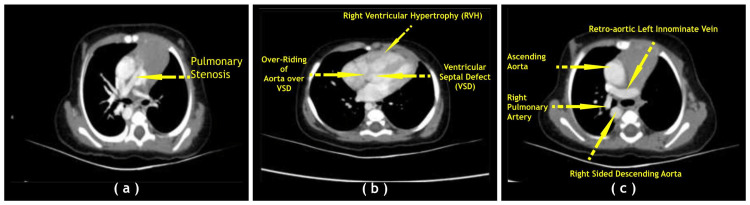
(a) CT axial image showing pulmonary stenosis and hypoplastic pulmonary arteries in a 6-month-old baby, who underwent primary Brock’s operation; (b) Axial image showing ventricular septal defect with over-riding the aorta as well as right ventricular enlargement; (c) Axial image showing retro-aortic left innominate vein and right-sided descending aorta. Image credits: Dr. Deepakkumar V. Mehta
